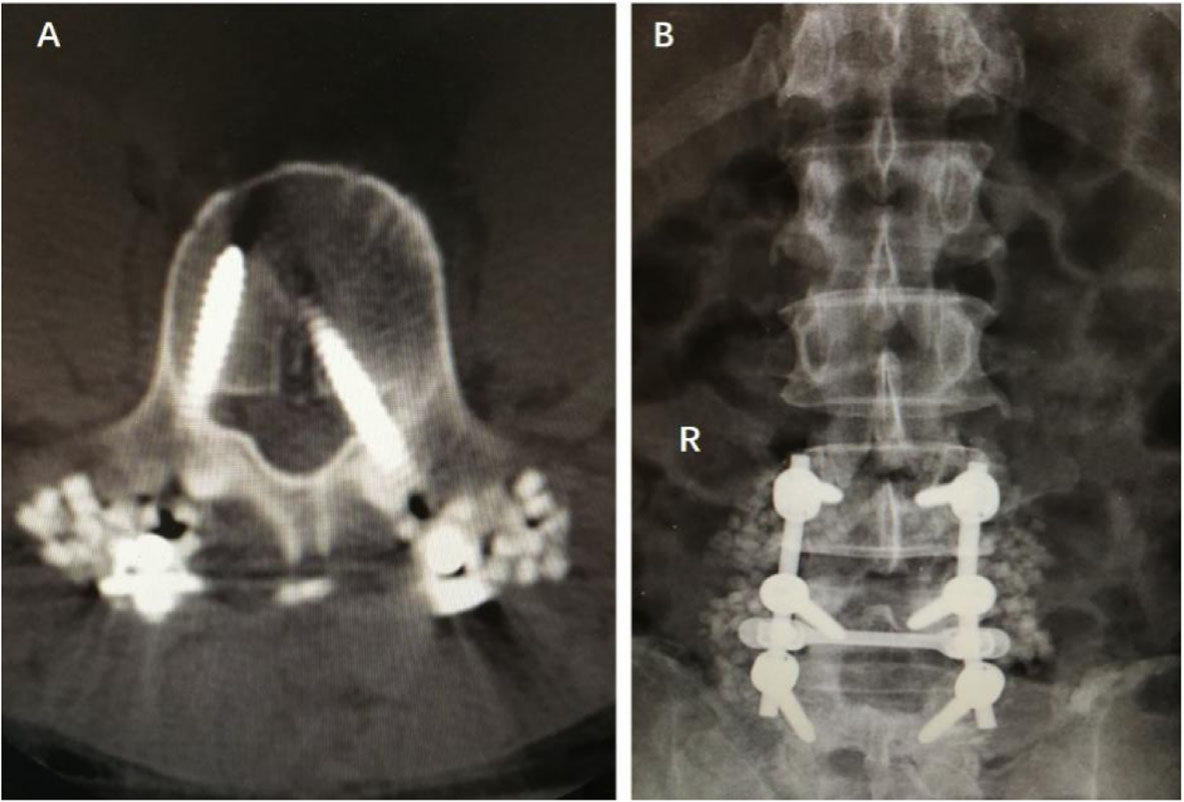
A 44-year-old male experienced numbness in his left dorso-phalangeal toe and had dorsiflexion and grade 2 muscle strength in his left ankle 1 day after an L4/5 discectomy and an L3–5 PLF. a CT scan showed that the left L4 pedicle screw had entered the spinal canal. b X-ray after the left L4 pedicle screw was adjusted during surgery. The patient’s symptoms (numbness, weakness) were relieved after reoperation
